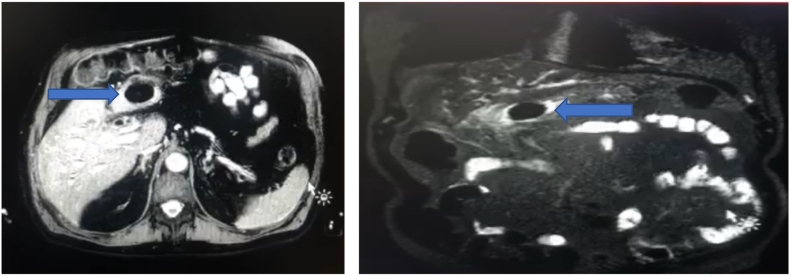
Left: MRI. Stone in gastric chamber. Right: MRI. Gall bladder adhesion to gastric chamber.
